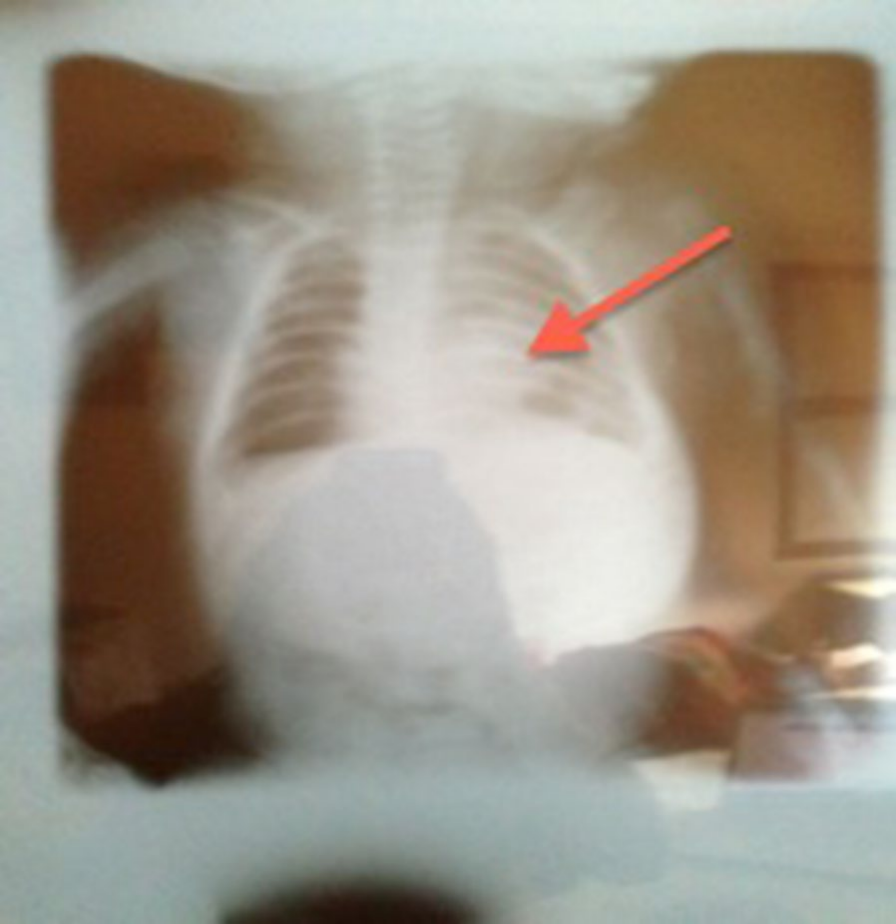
Chest X-ray of the patient taken within the first month of life, showing normal lung fields with distal displacement of the cardiac shadow.
